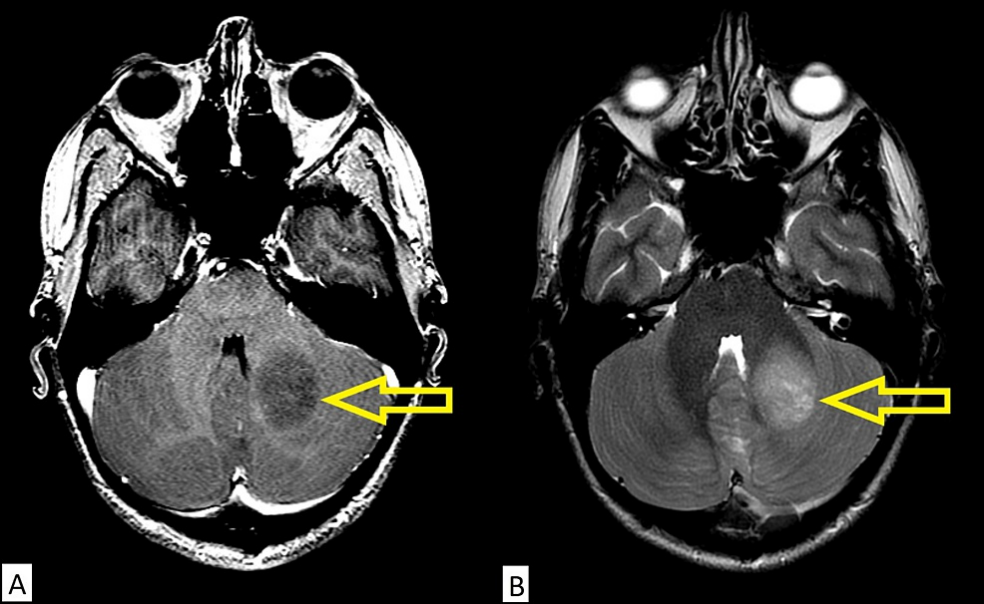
Preoperative MRI: (A) Gadolinium-enhanced T1-weighted and (B) T2-weighted axial images demonstrating a non-enhancing lobulated mass in the left cerebellum. MRI, magnetic resonance imaging
